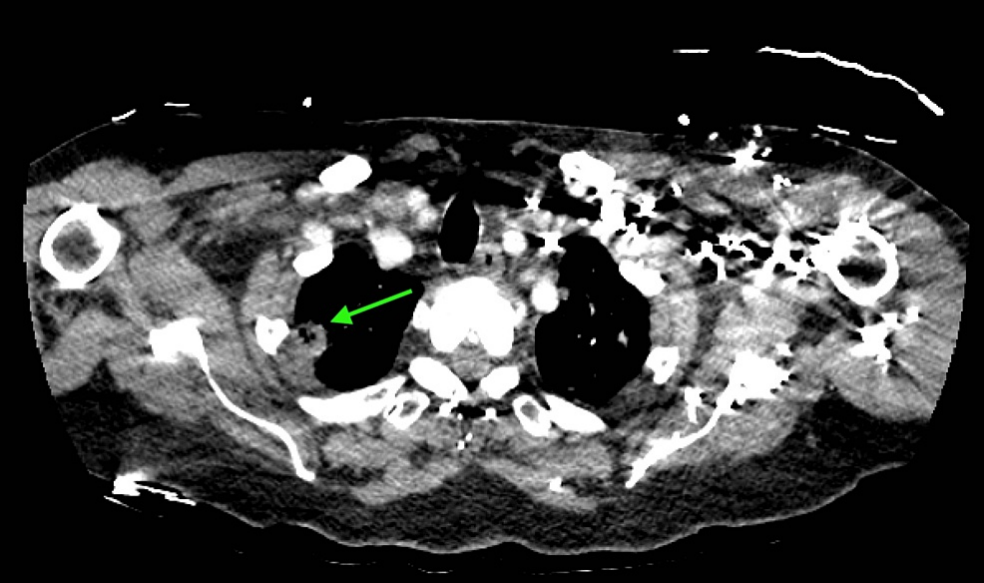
Apical lung CT, transverse cut, with cavitation suggestive of septic pulmonary emboli. CT: computed tomography
